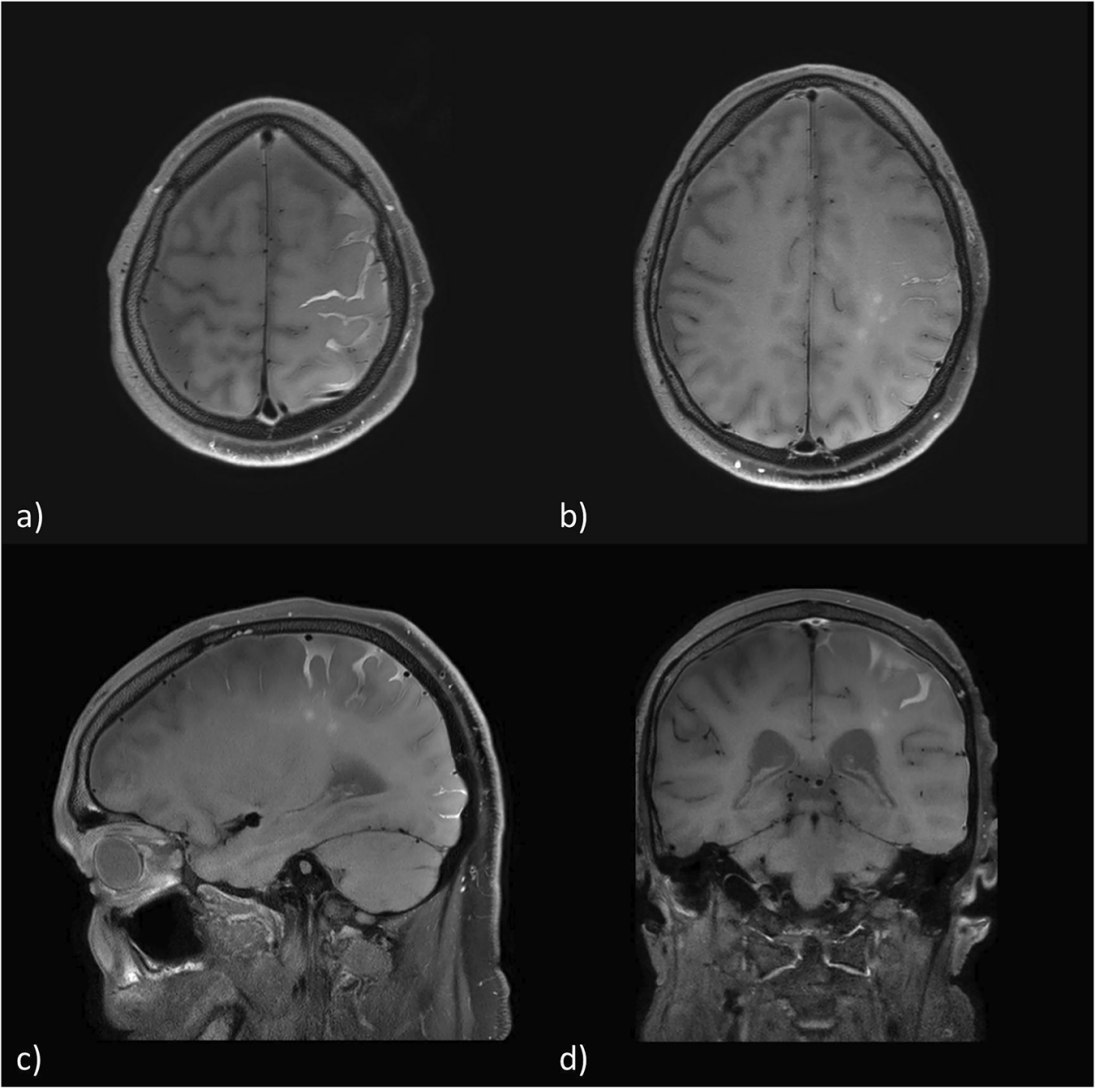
Follow-up MRI examination 3 h after GBCA administration in axial (a, b), sagittal (c) and coronal (d) reconstructions of three-dimensional T1-weighted sequence showing a diffuse subarachnoid (a, c, d) and parenchymal (b–d) GBCA enhancement. GBCA, Gadolinium-based contrast agent; MRI, Magnetic resonance imaging
